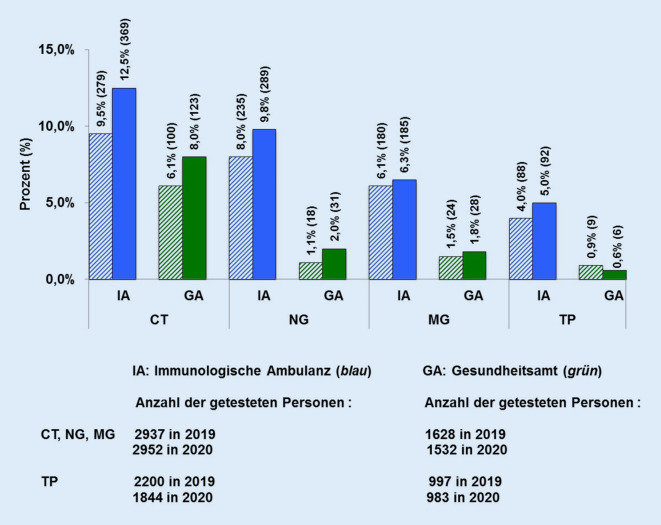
Bei den 18- bis 29-Jährigen waren 2020 die Infektionen mit CT (17,9 %), NG (11,4 %) und MG (7,6 %) am häufigsten. Um ein Drittel stiegen 2020 die NG-Infektionen bei den 30- bis 49-Jährigen auf 11,4 %. Seit einigen Jahren sind Änderungen in den Primärlokalisationen der STI zu beobachten: Die genitalen Infektionen mit CT und MG verdoppelten sich seit 2016 auf 52,6 % (2020). Die rektalen NG-Infektionen hingegen nahmen seit 2016 von 34,1 % auf 42,1 % zu. Bei MSM sind rund 55 % der STI rektal lokalisiert. NG wurden mit rund 30 % am häufigsten oral diagnostiziert.
